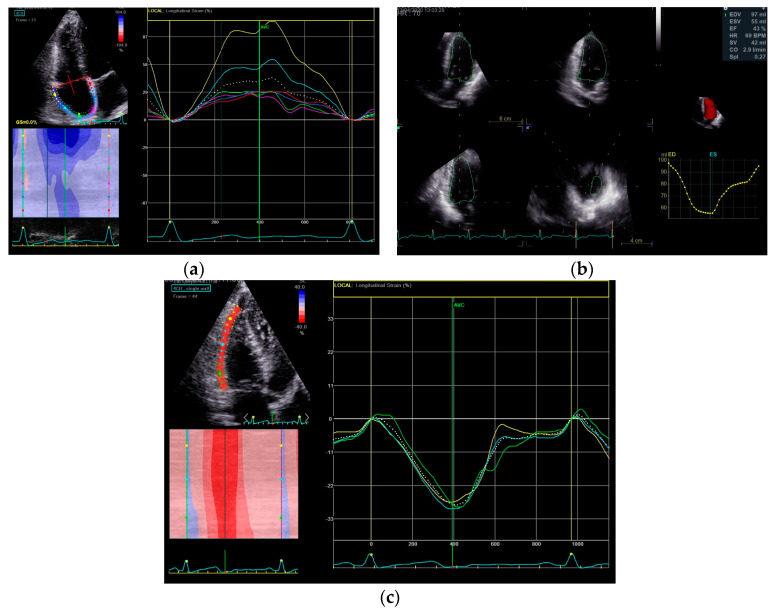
Novel echocardiographic indices of pulmonary hypertension prognosis: (a) RA strain; (b) 3D RV volume; (c) RV strain.
